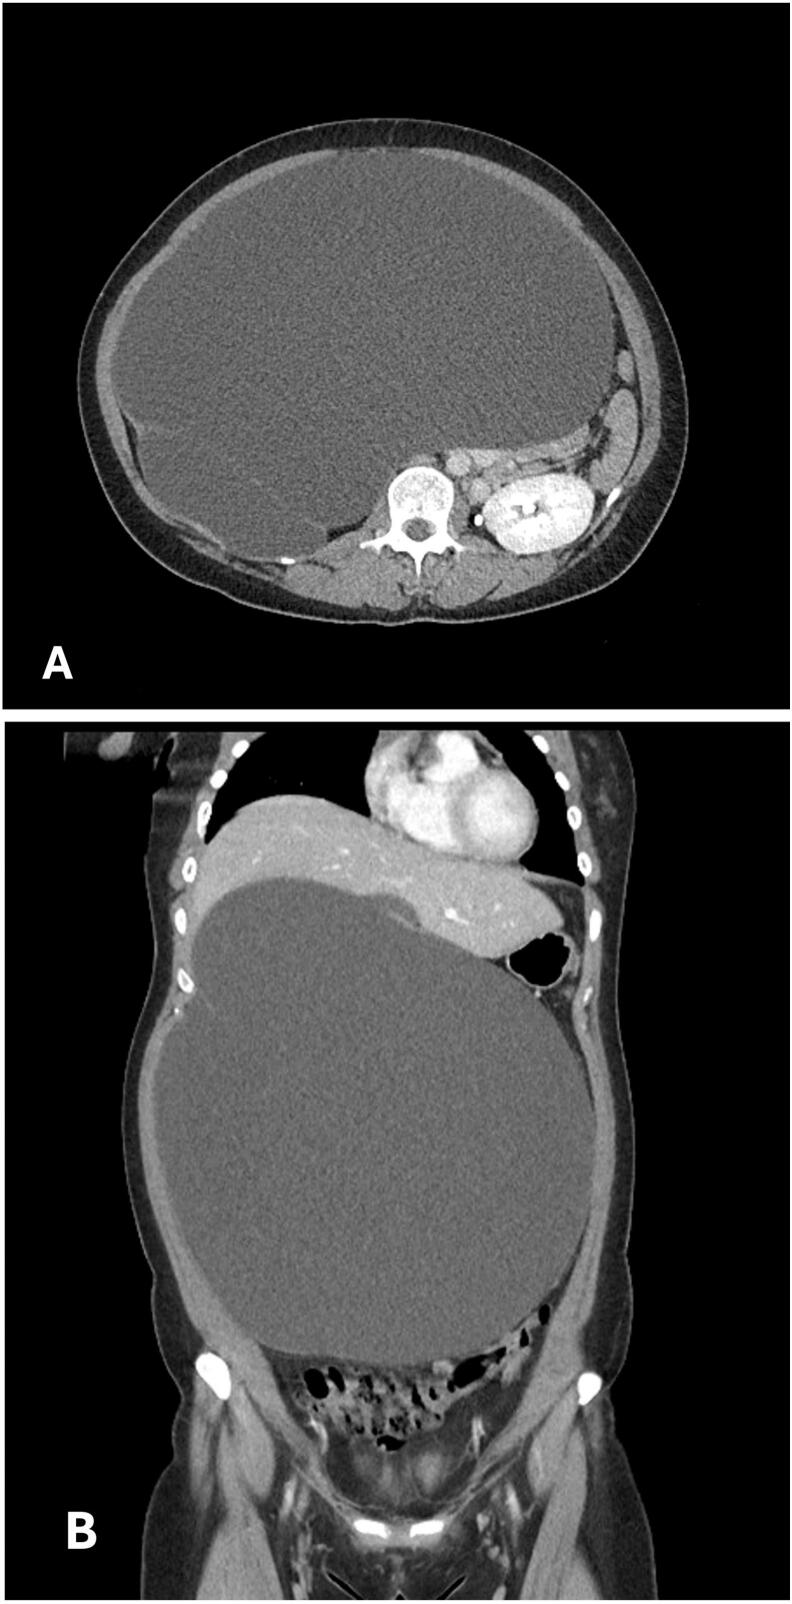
The axial (A) and coronal (B) views of an abdominal computed tomography scan reveal a large cystic mass occupying the entire abdominal cavity and displacing the bowel posteriorly, suggesting the possibility of an abdominal lymphatic malformation or an omental cyst.
